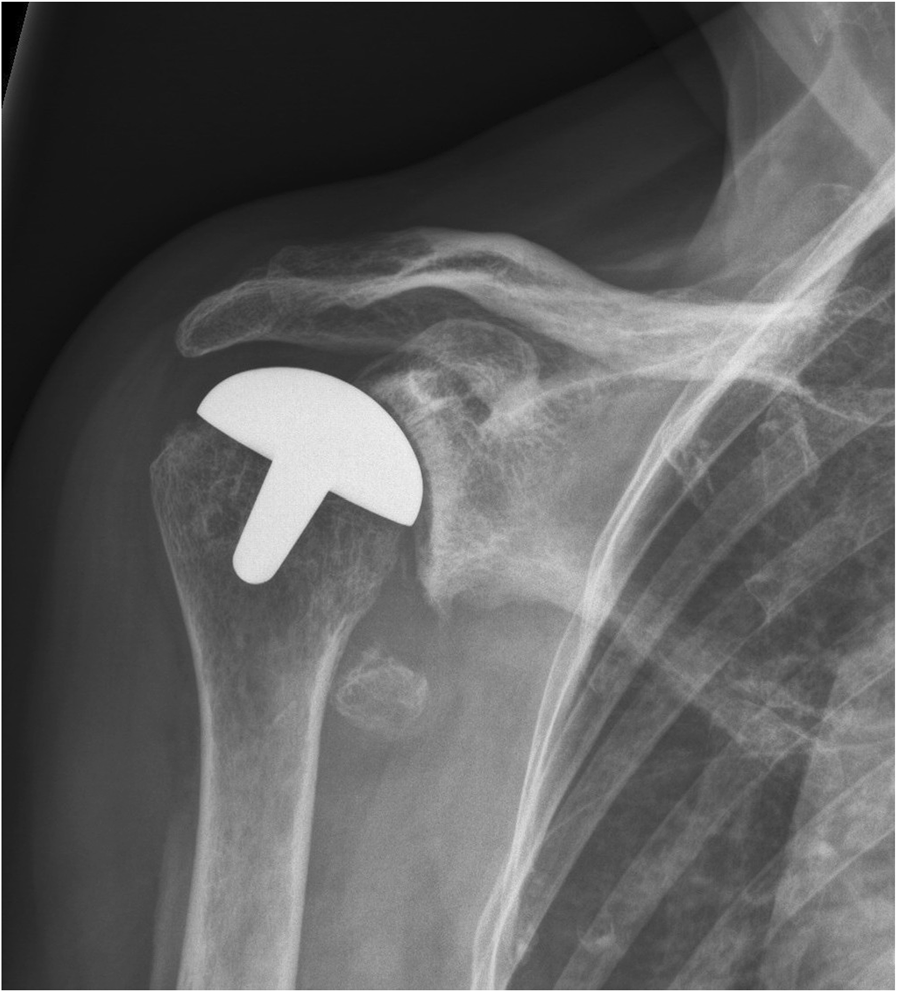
Post-implant AP radiograph where all observers classified the inclination as being in valgus
